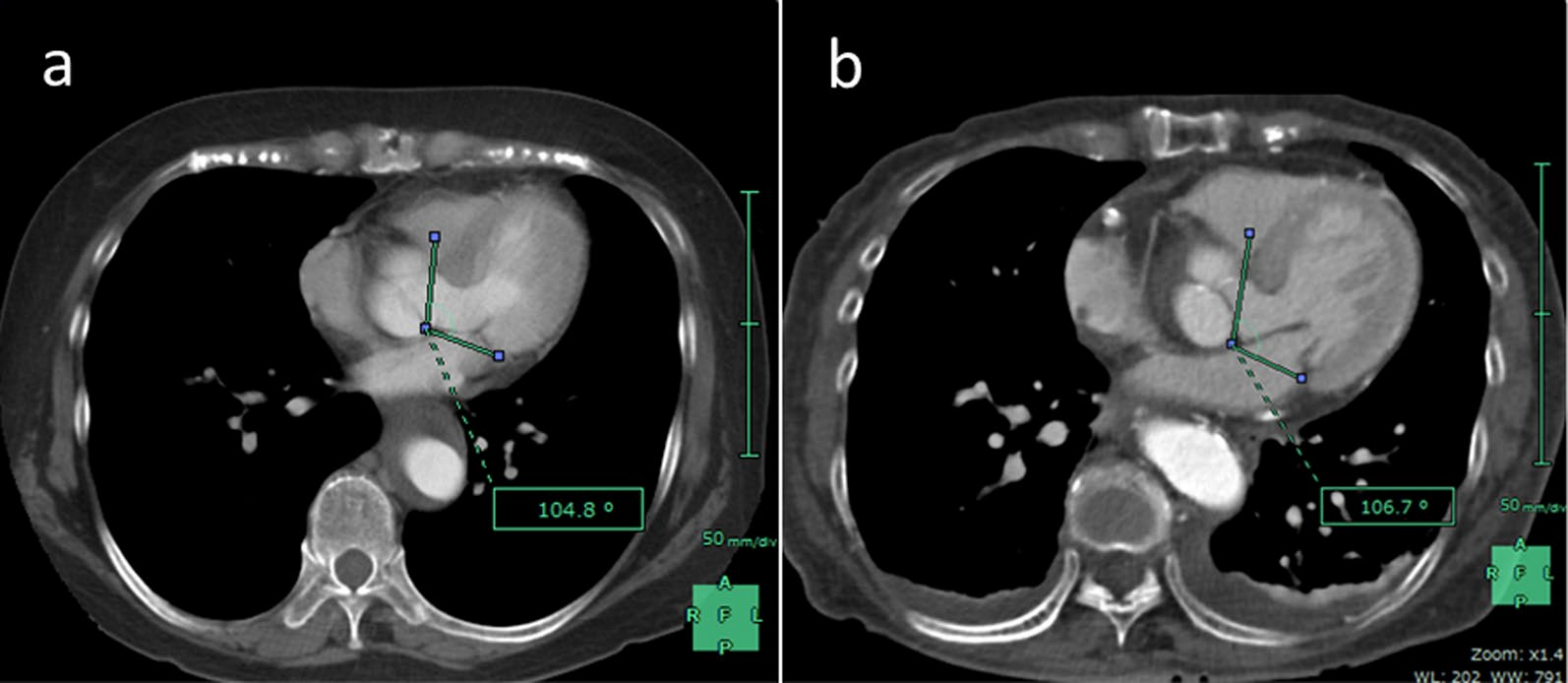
Narrow aorto-mitral angle detected in computed tomography. a Her past image before the onset of acute type A aortic dissection (at the onset of type B aortic dissection). b Preoperative image after the onset of acute type A aortic dissection. Both images have almost the same cross section
